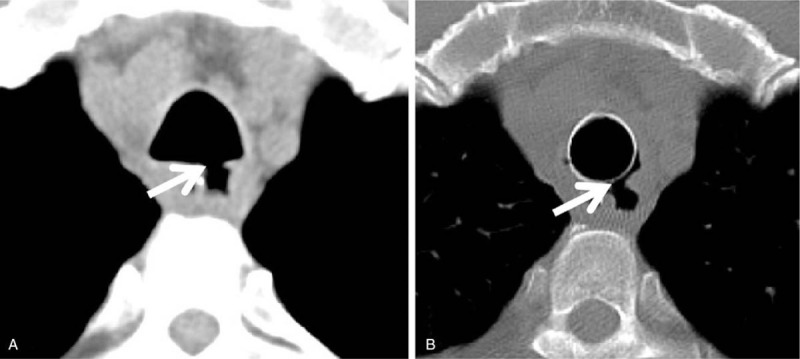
(A): Preoperative computed tomography image shows a high cervical tracheo-esophageal fistula (arrow); (B): the fistula has been completely blocked by the covered stent.
